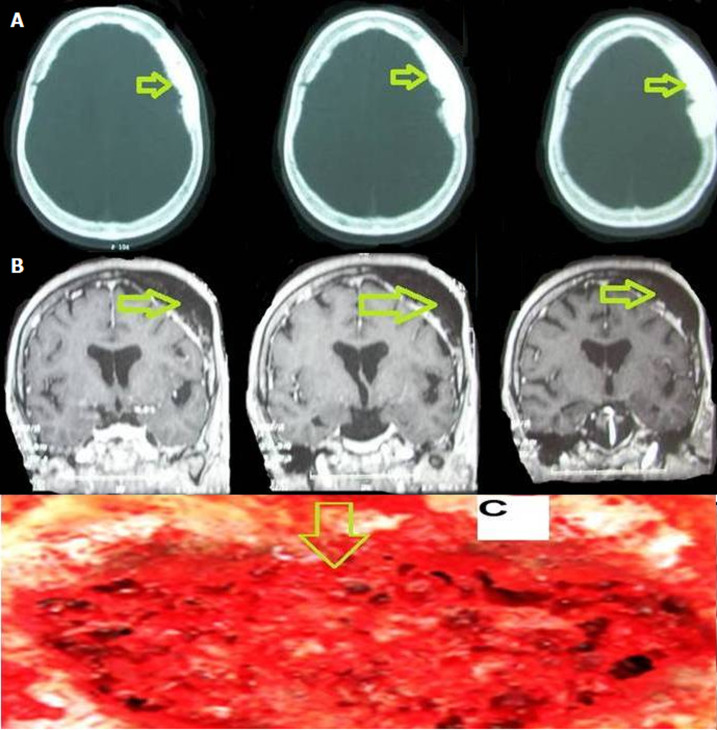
A) computed tomography (CT) of the head which demonstrated a mass with a bone density, which infiltrates the parietal bone; B) cerebral MRI in frontal section sequence T1 which demonstrated a mass lesion in parietal bone with low to intermediate T1 signal intensity; C) intraoperative image showing the lesion
